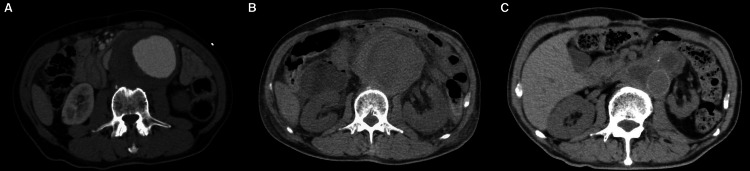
Abdominal CT on admission (A), day six (B), and postoperatively (C). Abdominal CT shows expansion of the abdominal aortic aneurysm from admission (A) to day six (B). Postoperative abdominal CT shows graft-replaced abdominal aorta and the decompressed duodenum (C). CT: computed tomography
